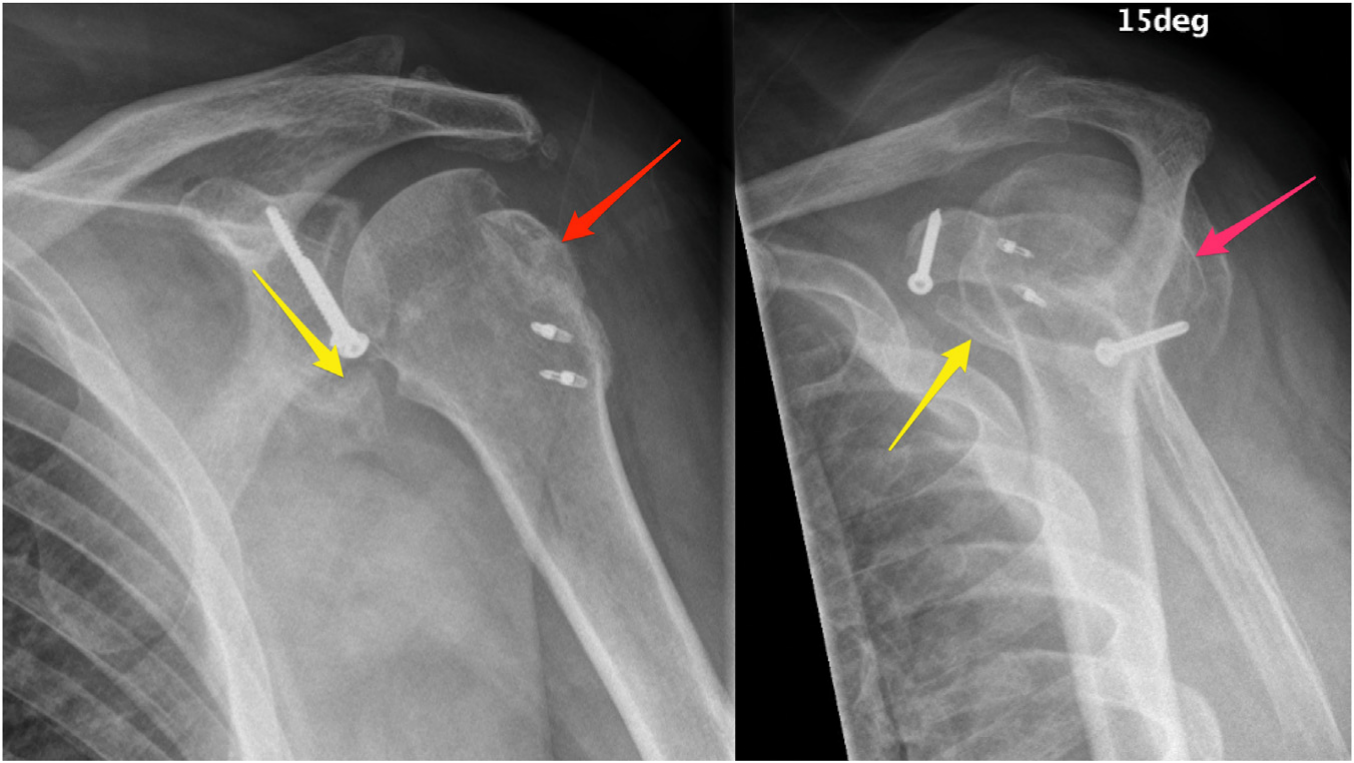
Radiography (2 views) from orthopedic surgery follow-up at 6 weeks postoperatively. *Red arrow* indicates greater tuberosity height loss and *yellow arrow* indicates minimal displacement of inferior glenoid fragment.
